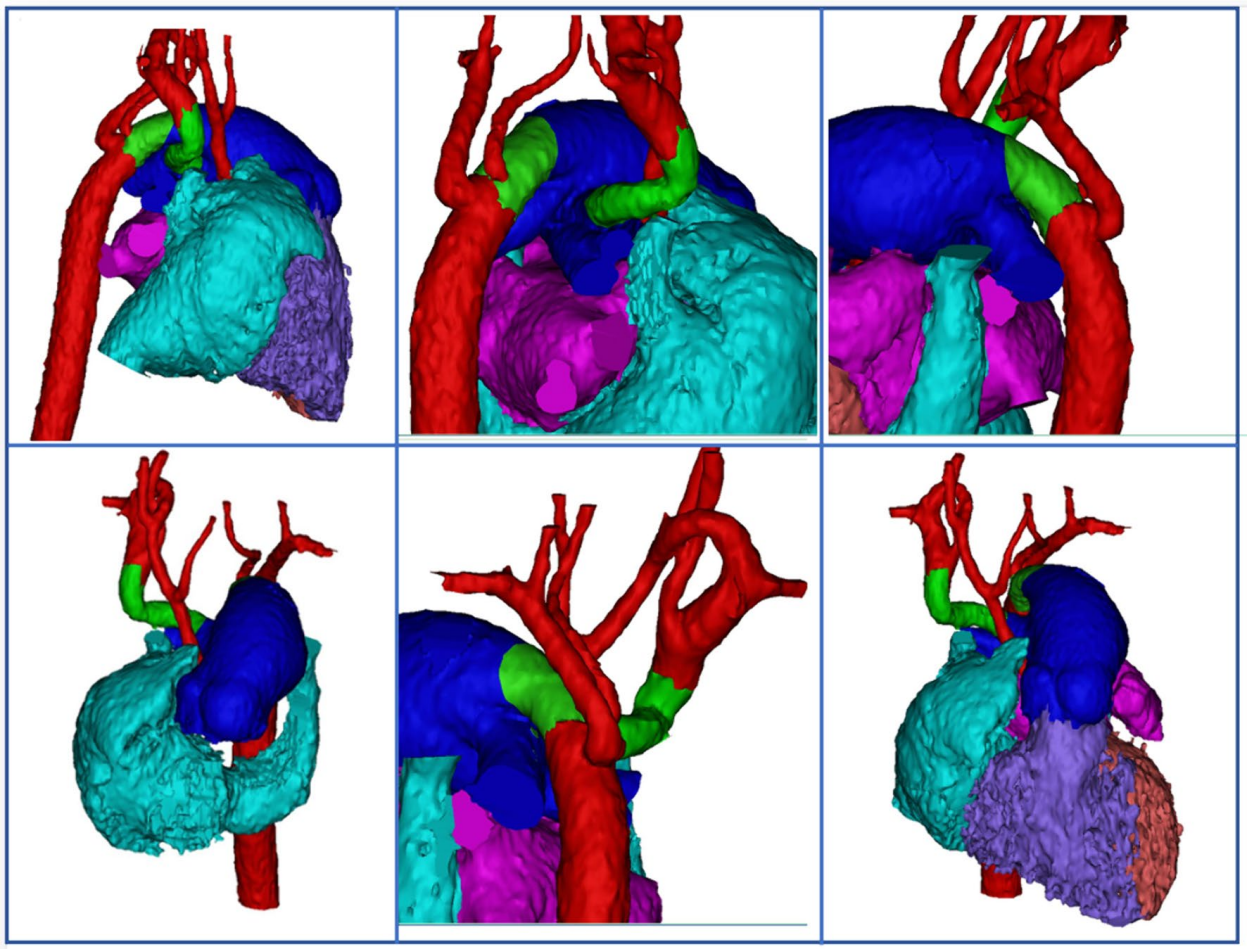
CT scan before the surgery: aortic atresia with a ventricular septal defect and interruption of the aortic arch type B
